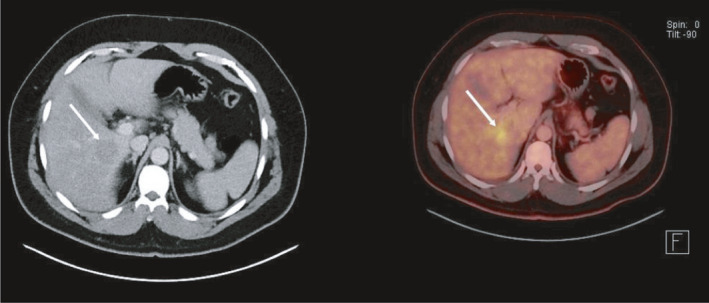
PET‐CT of liver shows a hypodense nodule in segment 5‐6 with heightened metabolic activity
